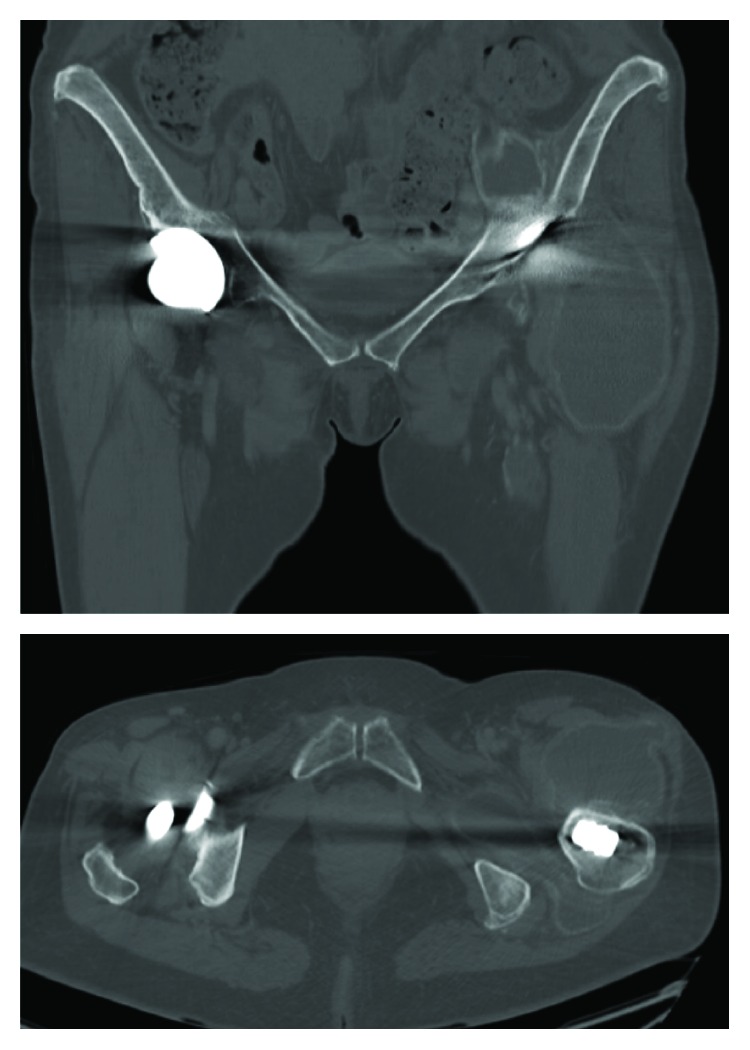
Frontal and axial views of the CT scan showing the important left periprosthetic collection.
